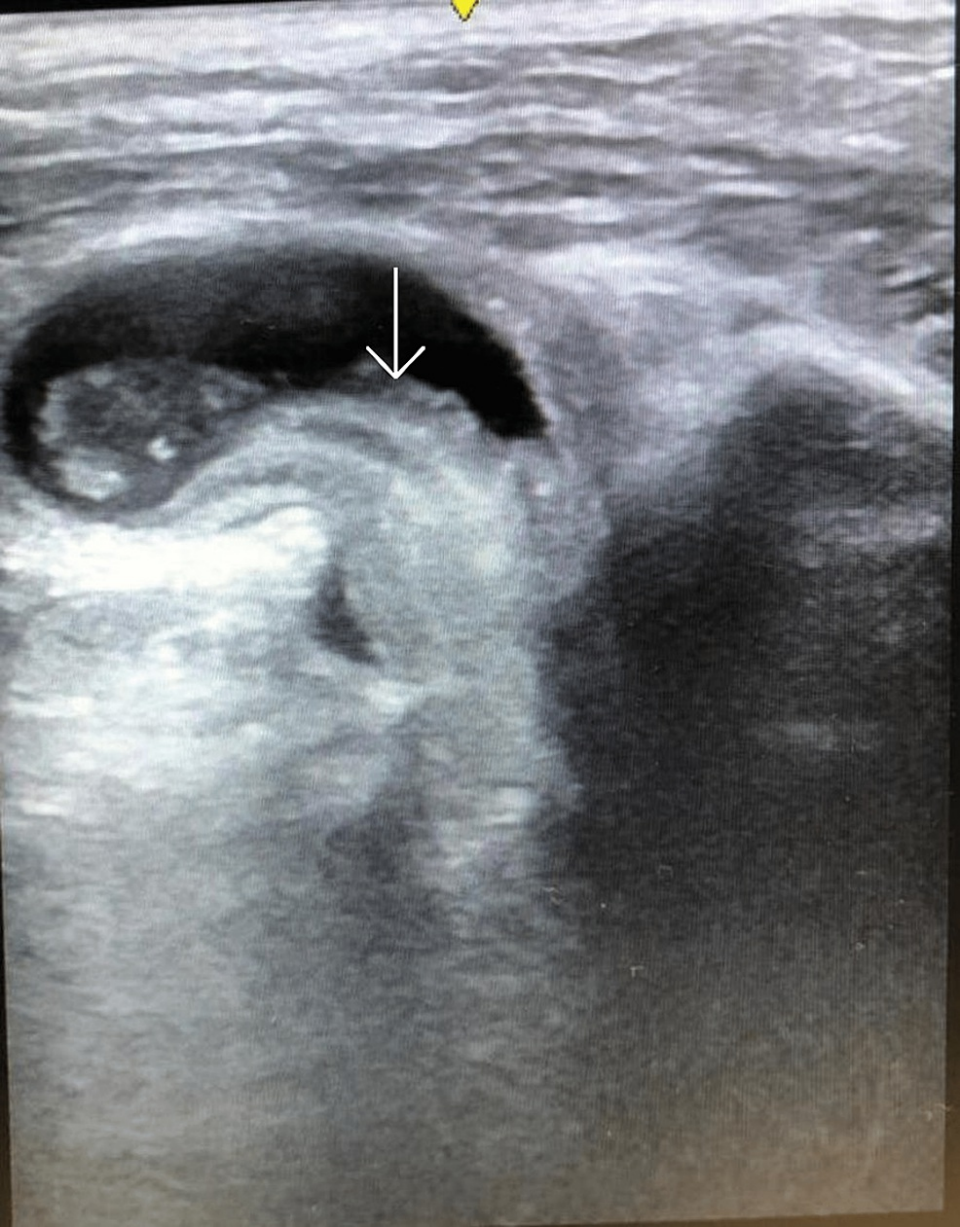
POCUS scan of the inguinal region (transverse plane) showing the obturator hernia with incarcerated loops of bowel (white arrow). POCUS: point-of-care ultrasound
